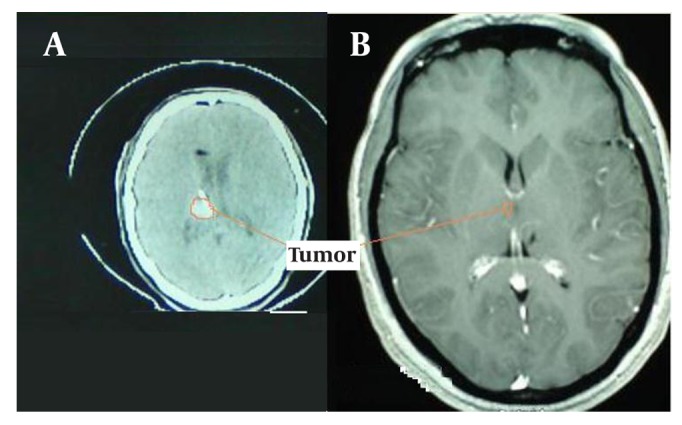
A Preoperative CT Scan from One Patient Undergoing SBT Treatment (B). Postoperative MRI from the Same Patient Nineteen Years Later (A)
